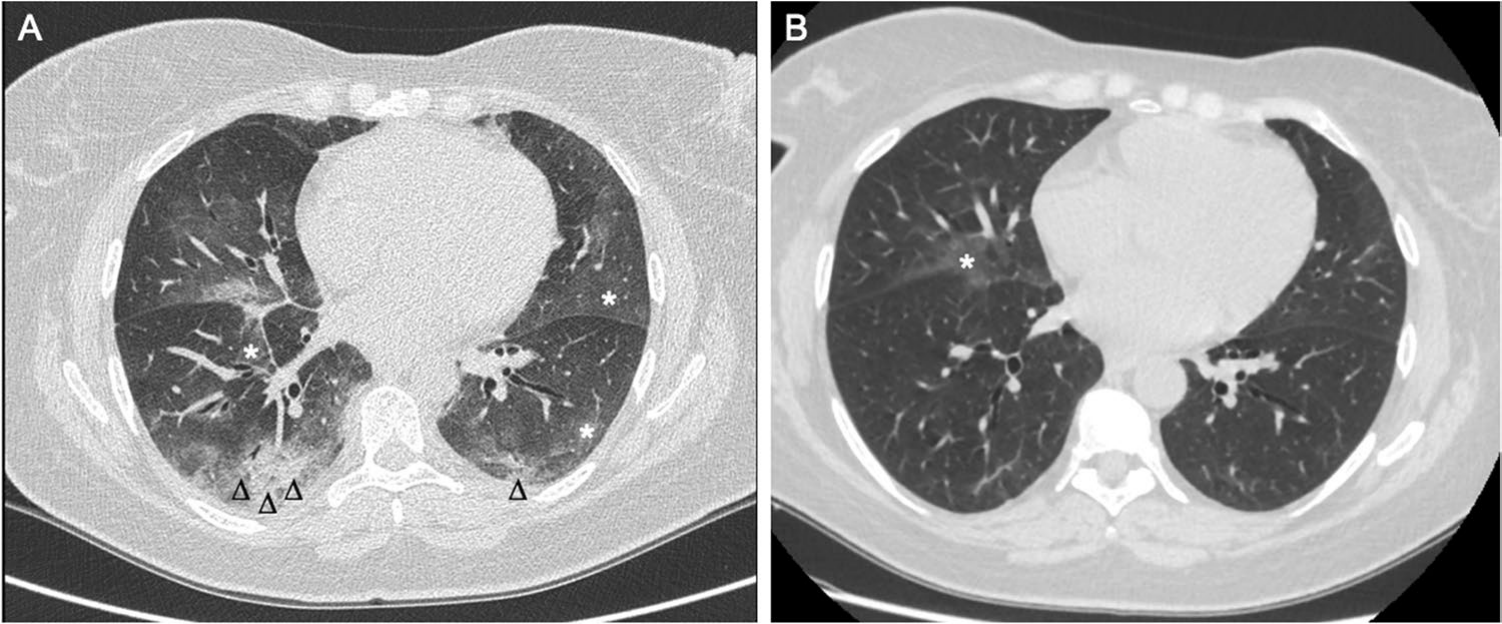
High-resolution computed tomography scans of a patient with rheumatoid arthritis-associated interstitial lung disease at baseline, starting abatacept treatment (A), and after 18 months of therapy (B). In A are detectable “ground-glass” opacities (asterisks) and pulmonary consolidations (arrowheads), while in B “ground-glass” opacities are significantly reduced and pulmonary consolidations disappeared
